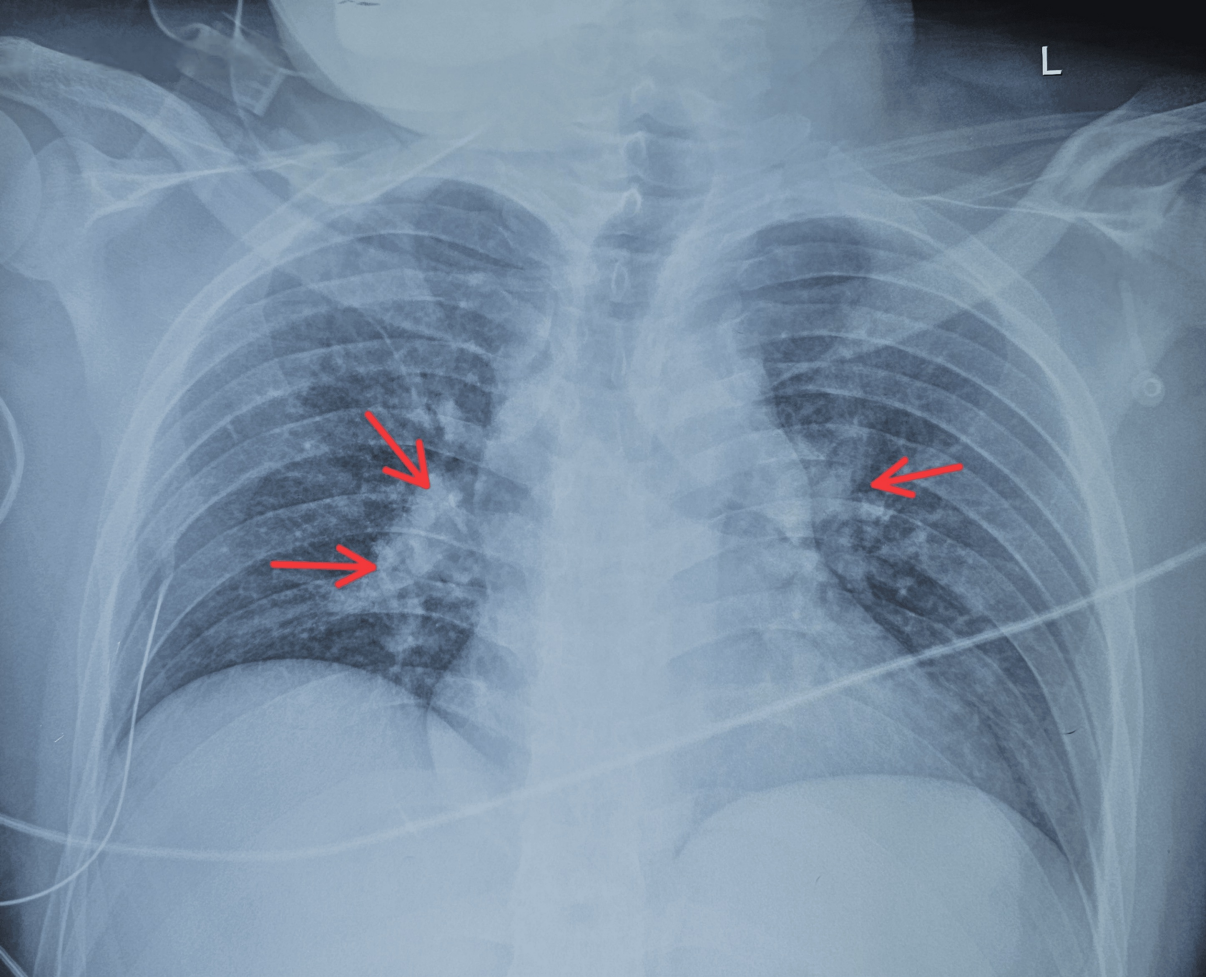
Chest X-ray showing bilateral perihilar haziness (red arrows)
